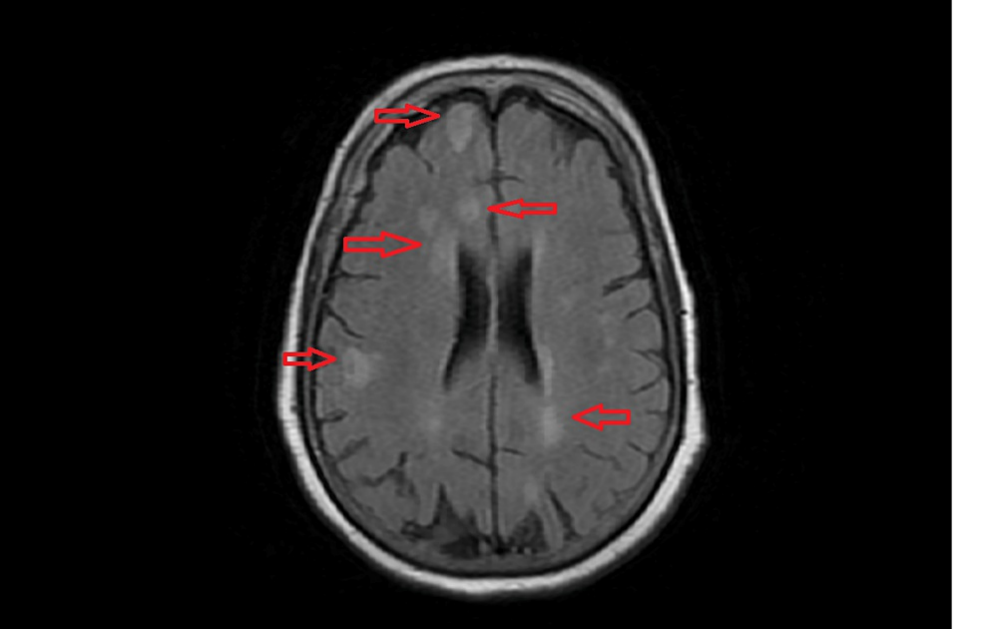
FLAIR image of the brain MRI of the patient showing multiple white matter hyperintensities suggestive of acute demyelinating disease FLAIR: fluid-attenuated inversion recovery; MRI: magnetic resonance imaging
